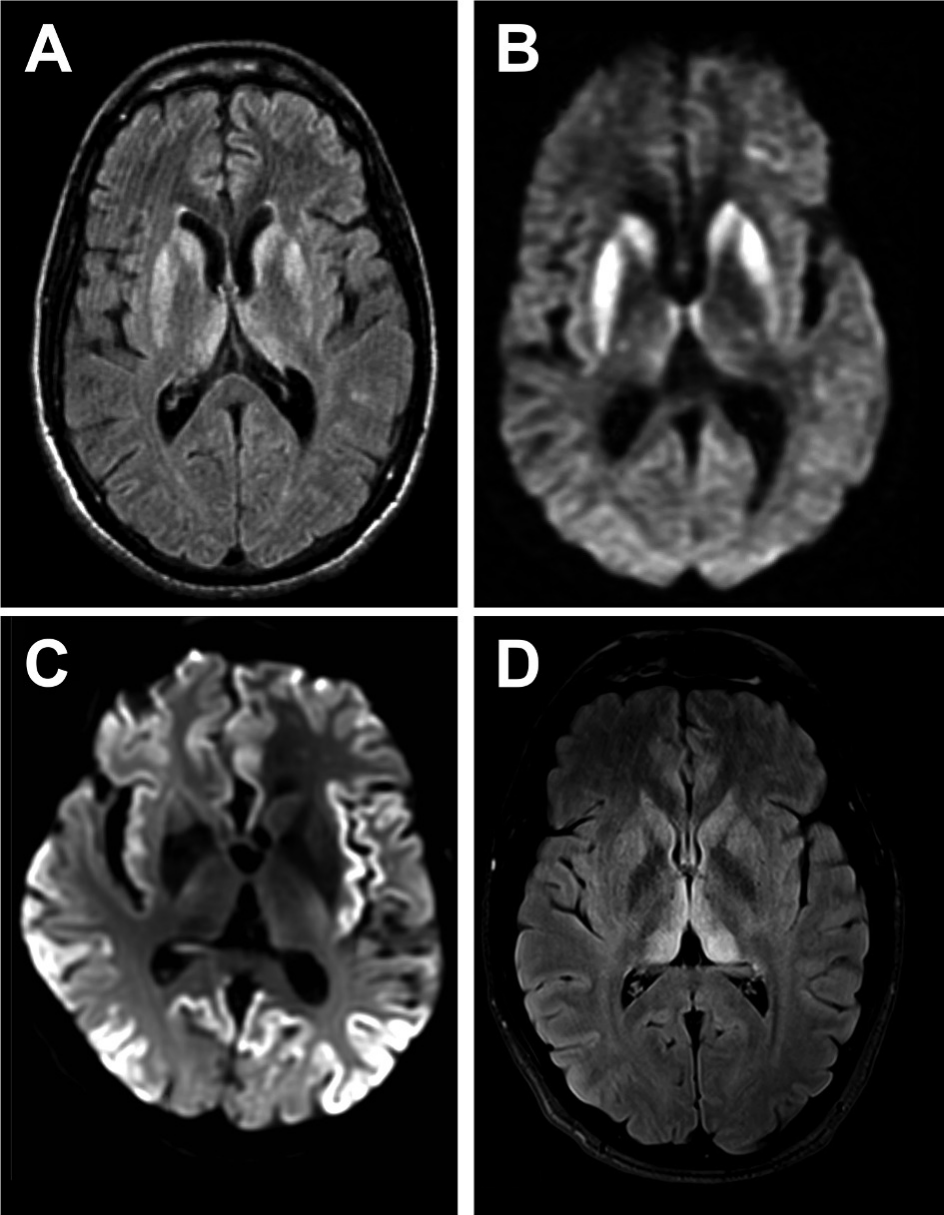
Typical MRI features of Creutzfeldt-Jakob disease (CJD). (A and B) Sporadic CJD showing typical basal ganglia signal return on fluid-attenuated inversion recovery (FLAIR) (A), which is more obvious on diffusion-weighted sequences (B). (C) Diffusion-weighted imaging sequence showing striking cortical ribboning with normal basal ganglia in sporadic CJD. (D) Variant CJD showing pulvinar sign on the FLAIR sequence.
